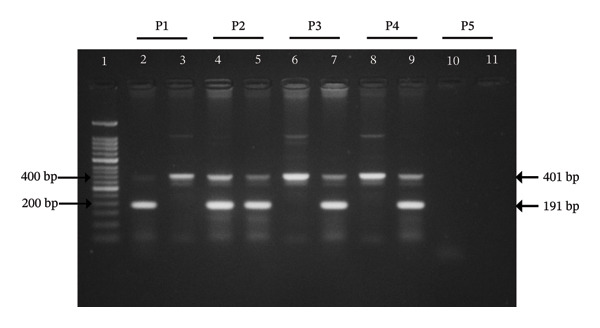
Allele‐specific PCR (AS‐PCR) analysis of the LDLR rs688 (C/T) polymorphism. A 191‐bp DNA fragment represents the presence of the corresponding allele, whereas the absence of amplification indicates the lack of that allele in the sample. A band at 401‐bp serves as a positive control. Lane 1 contains the 50 bp DNA ladder, and lanes 2–11 represent individual samples. Samples were run in pairs (P1–P5) with wild‐type and mutant‐specific forward primers. Each run also included the positive‐control forward and reverse primers which served as an indicator of DNA quality by producing a 401 bp band in all samples. Among the sample pairs, P1 denotes the homozygous CC genotype, P2 denotes the heterozygous CT genotype, P3 and P4 denote homozygous TT genotype, and P5 serves as the negative control. The PCR products were resolved on a 2% (w/v) agarose gel.
